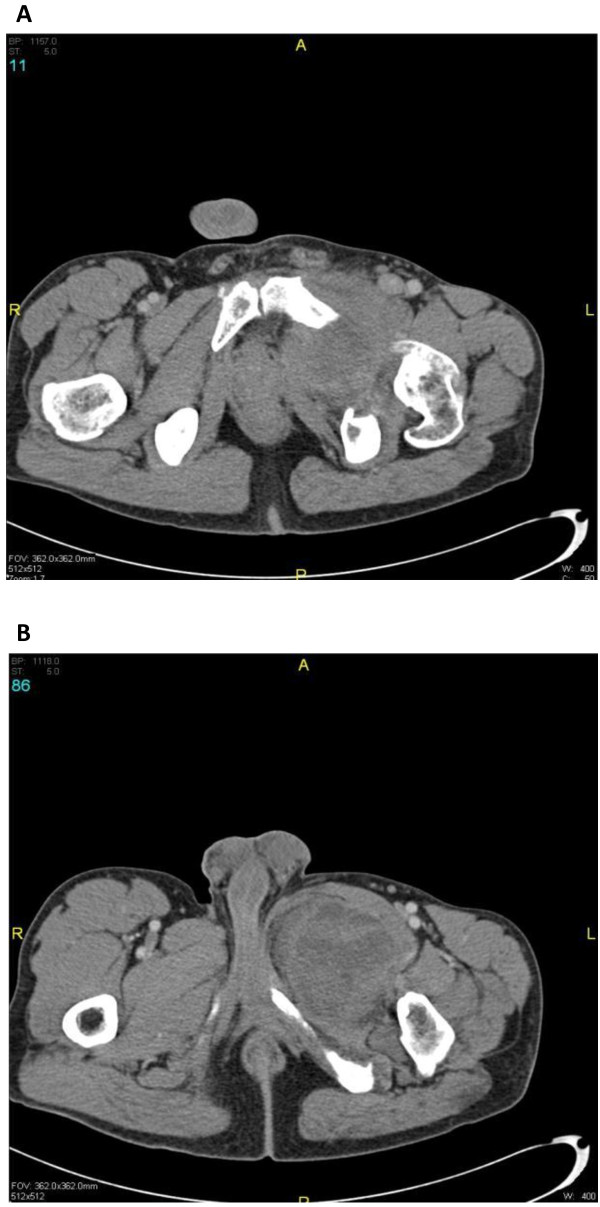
Pre-Operative CT Scan: a malignant peripheral nerve sheath tumor with extension through the obturator foramen in a 36 year-old man with Von Recklinghausen disease.
